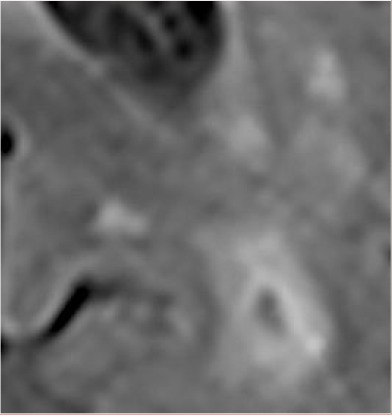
Enlarged perivascular spaces	Fluid-filled spaces that follow the typical course of a vessel through gray or white matter. Found in the basal ganglia or centrum semiovale. Similar signal intensity with CSF; increased T2-weighted signal decreased signal on FLAIR and T1-weighted MRI.	Enlargement of the anatomical compartment between vessel walls and the glia limitans of the blood–brain barrier; may reflect impaired interstitial fluid drainage from the brain associated with structural and functional consequences of CSVD. When located in the basal ganglia, EPVS are associated with arteriolosclerosis. EPVS in the centrum semiovale appear to be related to CAA.
